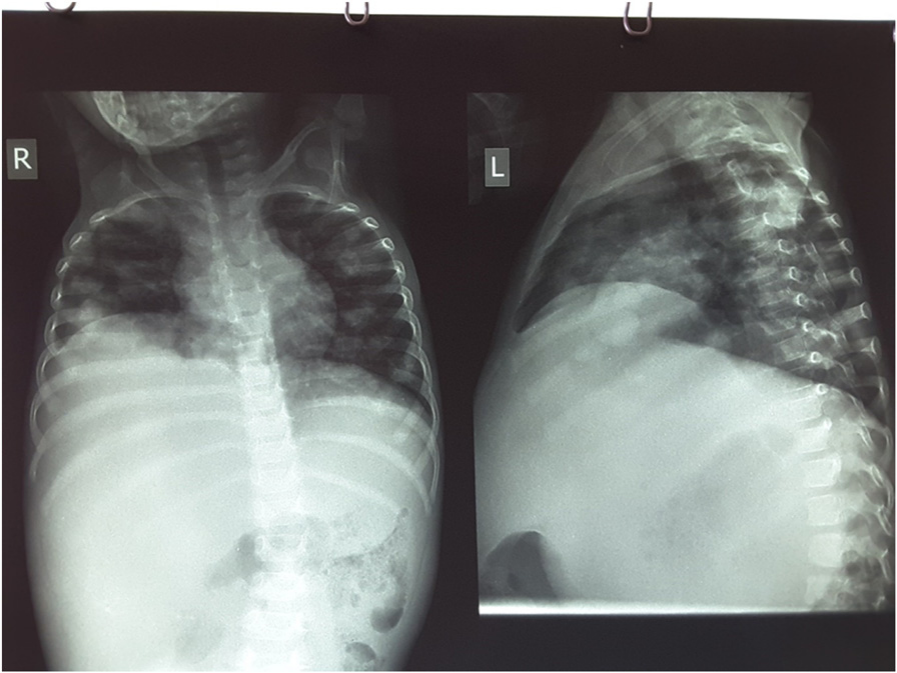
Plain chest X-ray showing cannonball metastases
